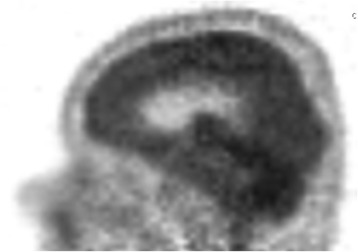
FDG-PET showing increased uptake in infratentorial regions, and partially on brain convexity
